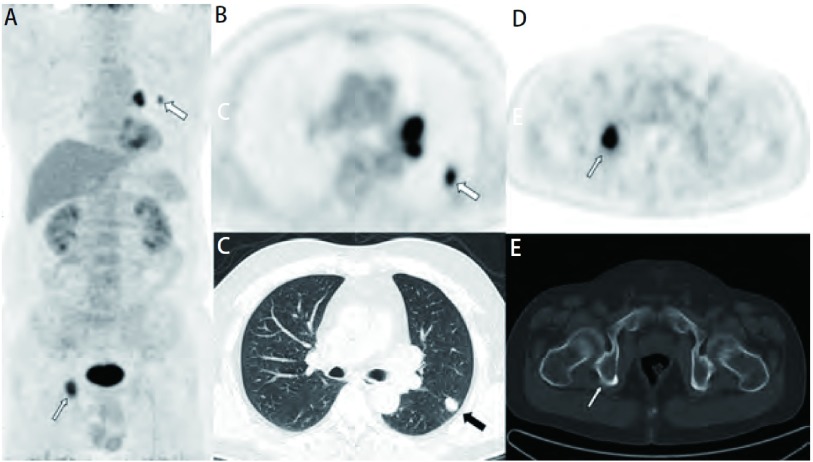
男，52岁，左肺一枚结节性病灶，CT未见典型恶性征象，PET示异常浓聚（A、B、C，粗箭头），SUVmean：3.5，按照诊断标准初步诊断为可疑恶性，但全身PET/CT发现该病例伴有同侧肺门肿大淋巴结和右侧坐骨的团块状异常FDG浓聚（A、D、E，细箭头），修正诊断为肯定恶性，最终经活检证实为小细胞肺癌。 A male patient, 52 years old, CT demonstrates a nodule in his left lung but not seemed as malignancy. A hypermetabolic lesion with SUVmean value as 3.5 was identified on PET image (A, B, C, crude arrow). Preliminary diagnosed as probable malignancy. Whole body PET/CT showed FDG accumulate in ipsilateral hilar lymph nodes and right ischium (A, D, E, fine arrow). Modified diagnosis as affirmative malignancy, and ultimately confirmed by biopsy of small cell lung cancer.
